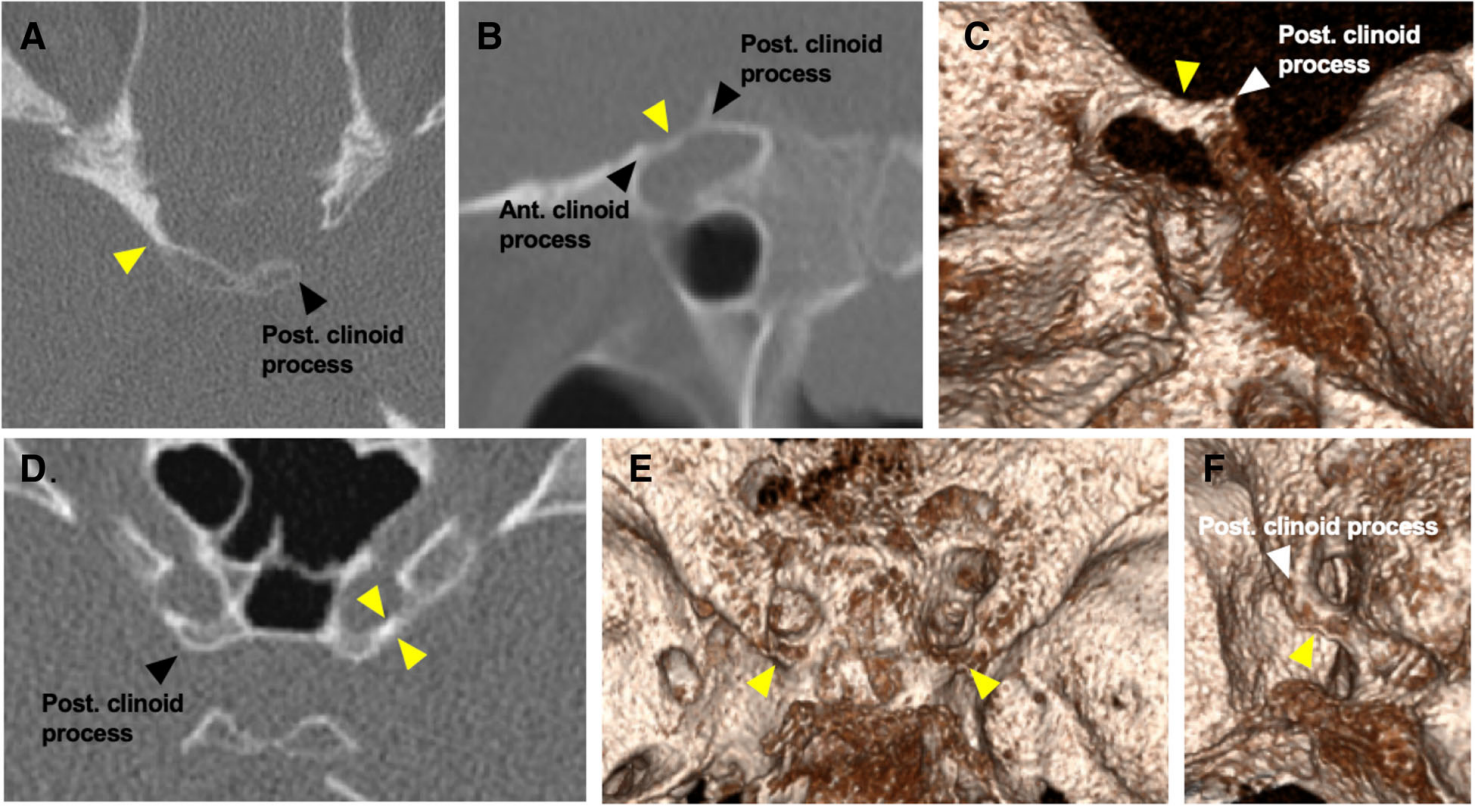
Mineralised caroticoclinoid and interclinoid ligaments. Axial (a), sagittal oblique (b), and 3D (c) volume reconstruction demonstrating a complete interclinoid ligament on the right (yellow arrowhead). Axial CT (d) and 3D (e) volume reconstructions demonstrating bilateral complete caroticoclinoid ligaments (yellow arrowheads). f 3D reconstruction demonstrating the right-sided complete caroticoclinoid ligament (yellow arrowhead)
